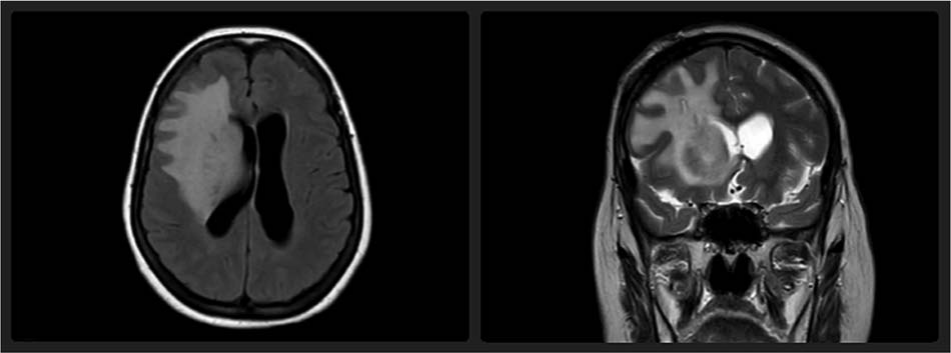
Axial FLAIR and coronal T2-weighted MRI performed three weeks after catheter removal demonstrate a notable reduction in the previously observed hyperintensity in the right frontal lobe, indicating partial resolution of the leukoencephalopathy.
